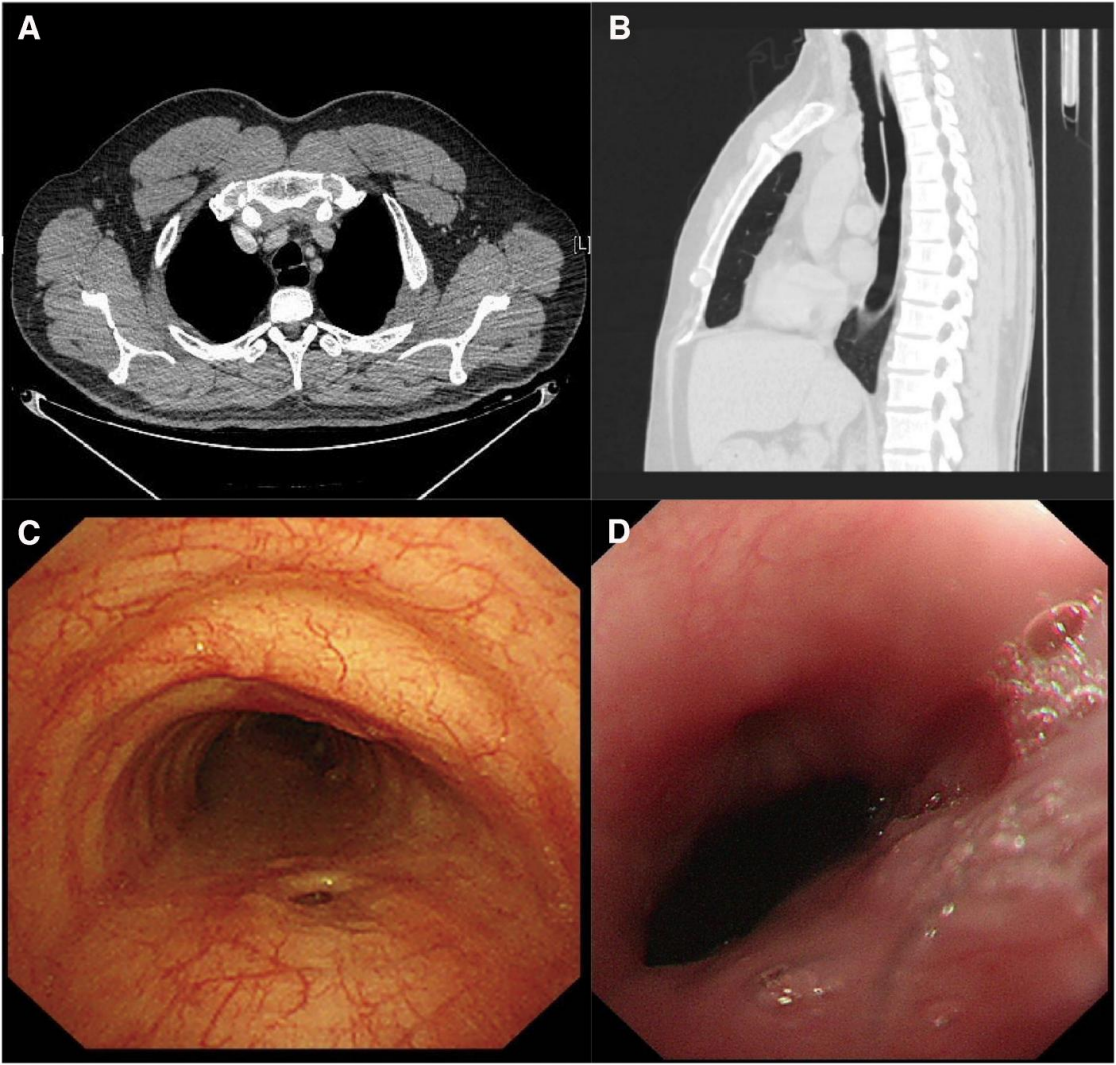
(A) Axial chest CT scan of the patient shows a communication between the trachea and oesophagus. (B) Coronal chest CT scan of the patient shows a communication between the trachea and oesophagus. (C) Endoscopic examination of the patient shows a fistula at the membranous portion. (D) Oesophagoscopic examination of the patient shows an oesophageal fistula.
